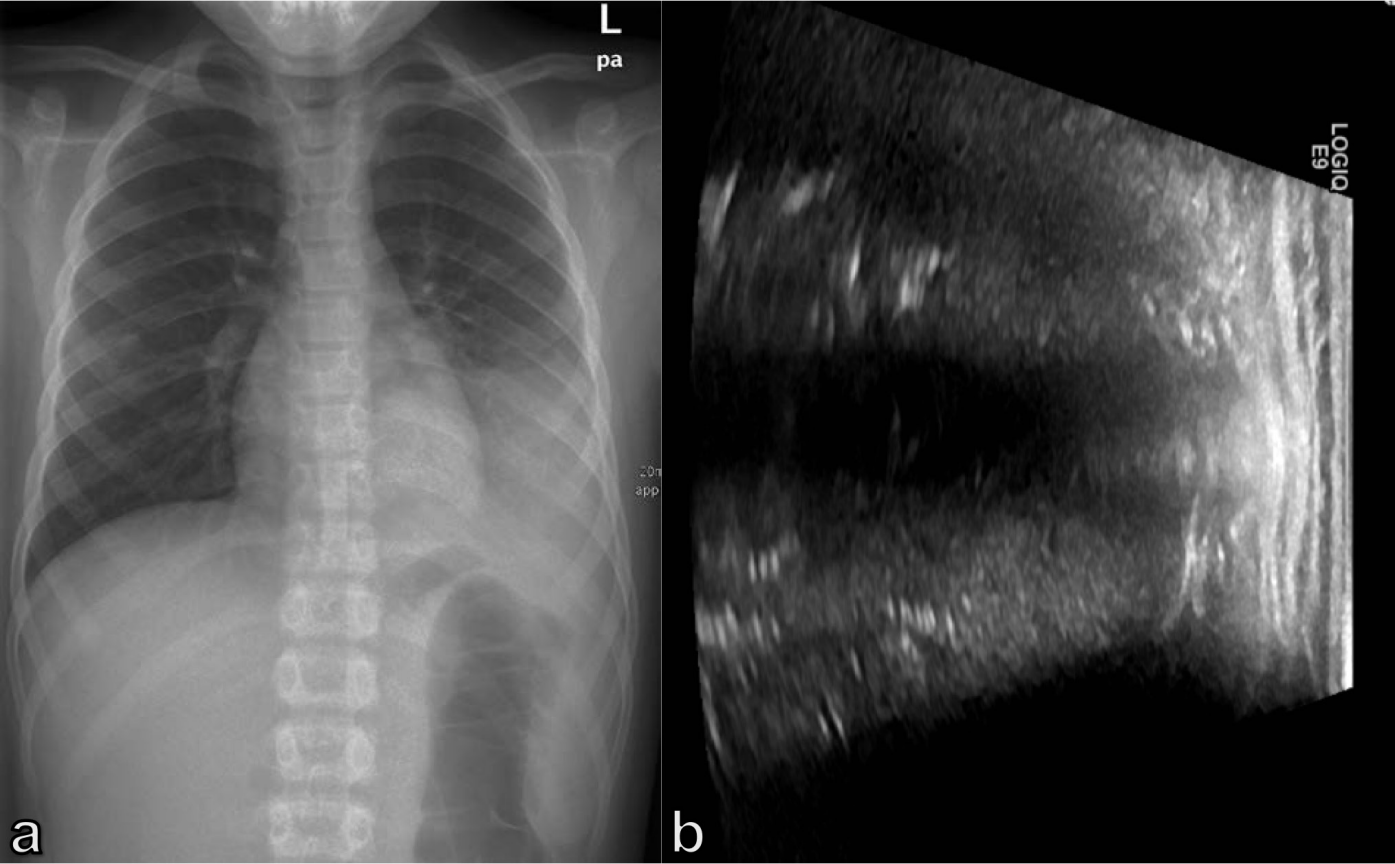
(a) CXR of a 9-year-old boy with dyspnea and pneumonia and (b) corresponding ultrasound image of the pneumonia, depicting the consolidation of the lung parenchyma with punctiform hyperechogenic spots that correspond to the air bronchograms. CXR, chest X-ray.
